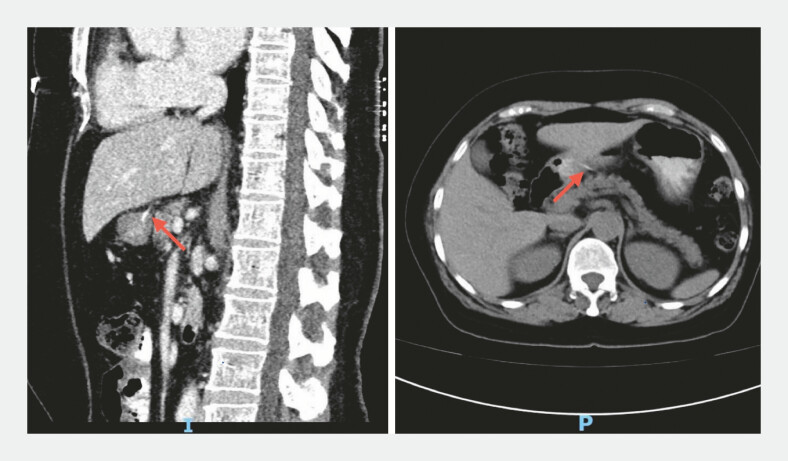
Contrast-enhanced computed tomography showed high-density linear shadows of the antrum and penetrated the stomach wall into the abdominal cavity.
